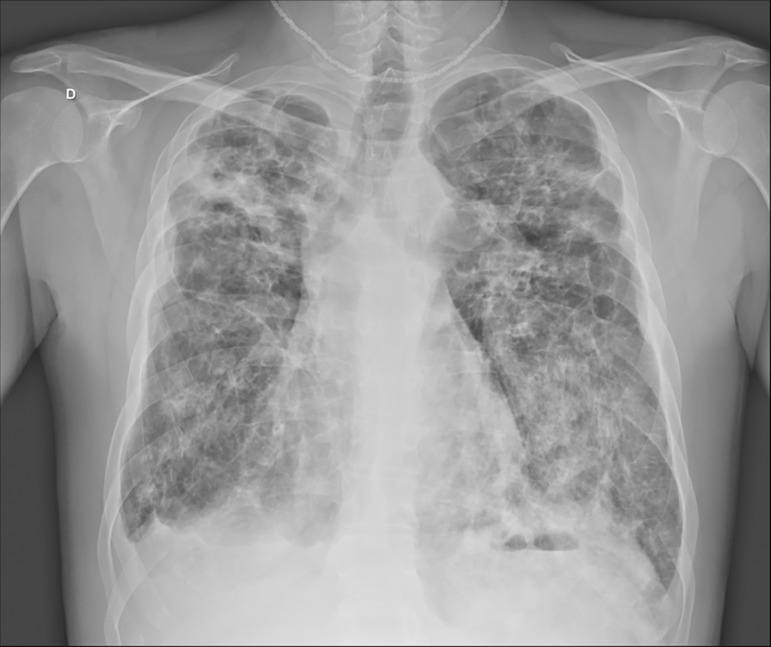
Chest X-ray at hospital admission, showing areas of old fibroatelectasis
